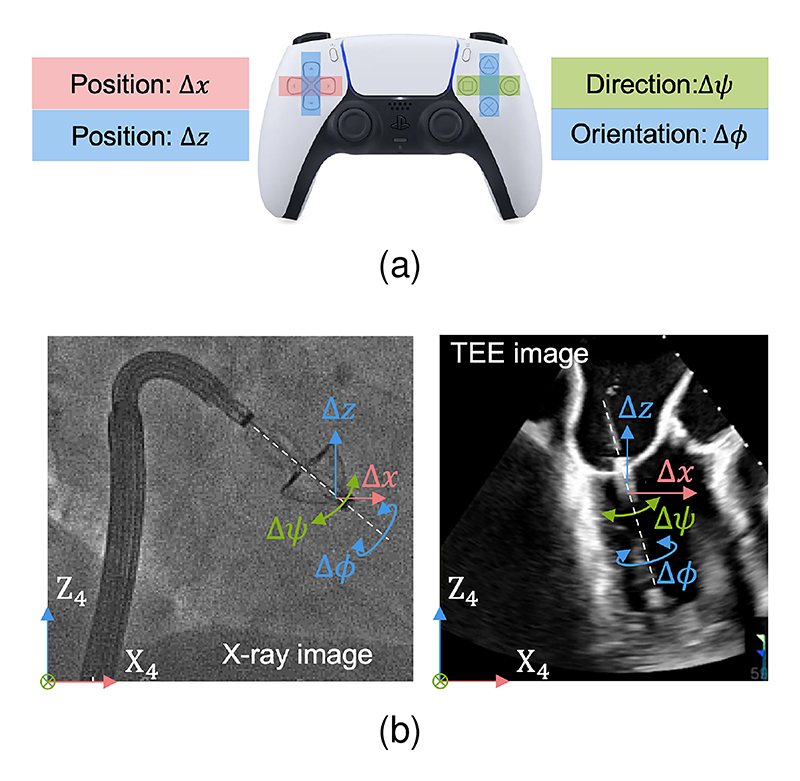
Independent control strategy based on registered images (a) Command assignments on the PS5 controller. (b) Clip response in registered image coordinates: X-ray (left, from [30], CC-BY) and ultrasound (right, from [31], CC-BY).
